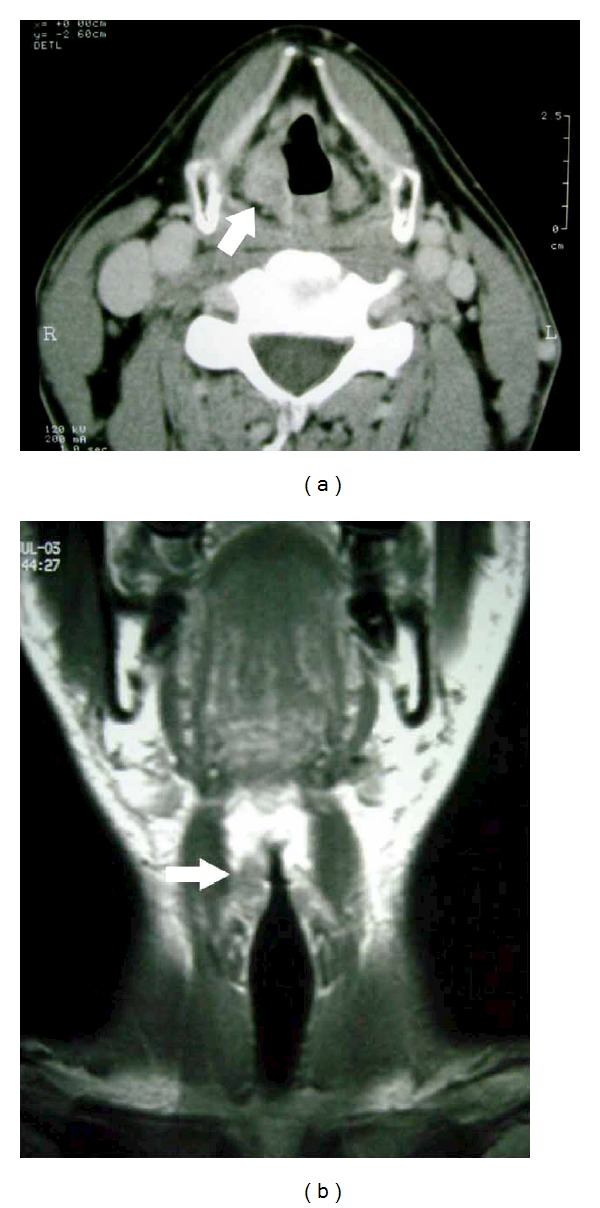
CT-scan (a) and MRI (b) showing a swelling of the right false cord (arrows) with involvement of the paraglottic space and without a sign of significant lymphadenopathy.
